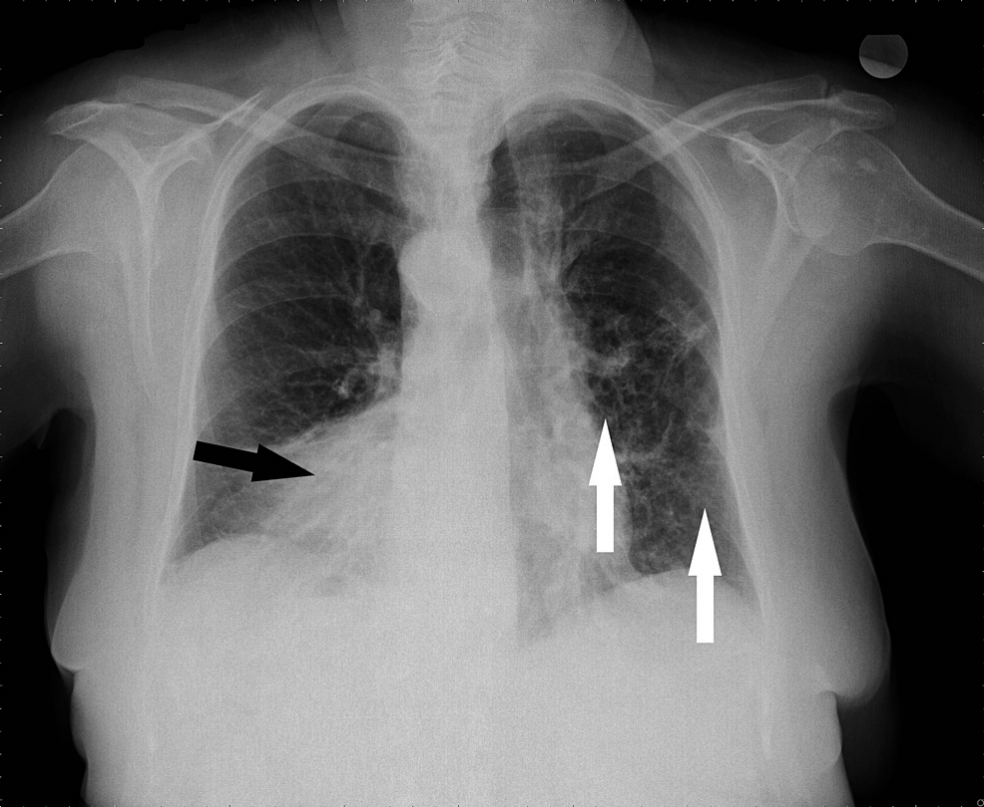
Chest X-ray. Chest X-ray in the emergency department showing the cardiac apex, the aortic arch, and stomach bubble on the right side, suggesting situs inversus. The black arrow indicates dextrocardia. There are confluent, cyst-like structures on the left lower lung zone, suggesting the presence of bronchiectases (white arrows).
